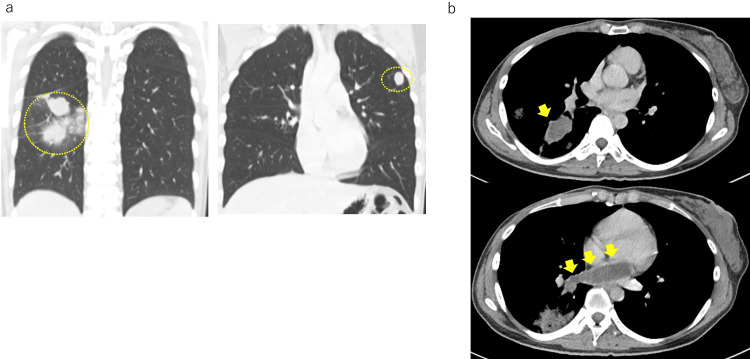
Computed tomography (CT) image of the metastatic lesion (a) Bilateral pulmonary metastases. (b) Tumor invasion into the pulmonary veins and progression within the vasculature
